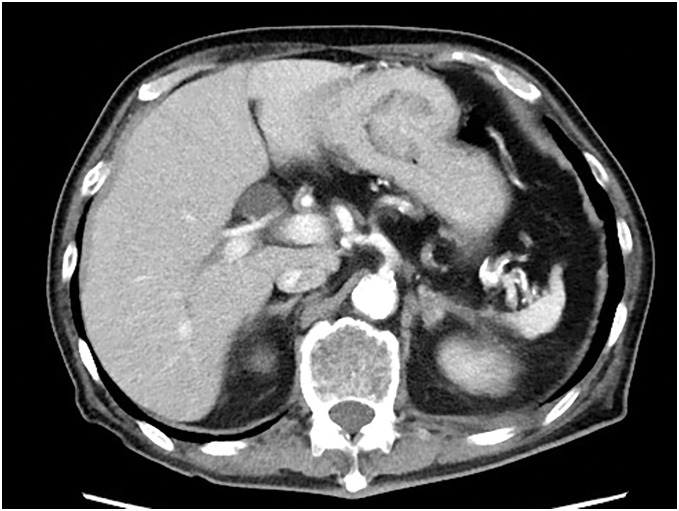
An abdominal CT scan showed a tumor with no serosal invasion, no lymph node enlargement, and no distant metastasis.
